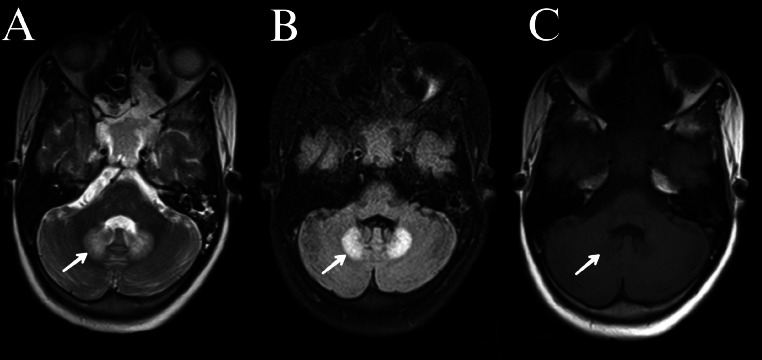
MRI images of the brain at the level of middle cerebellar peduncle shows T2 (A) and FLAIR (B) hyperintensity (indication with white arrow) of bilateral dentate nuclei with T1 (C) hypointensity (indication with white arrow).
